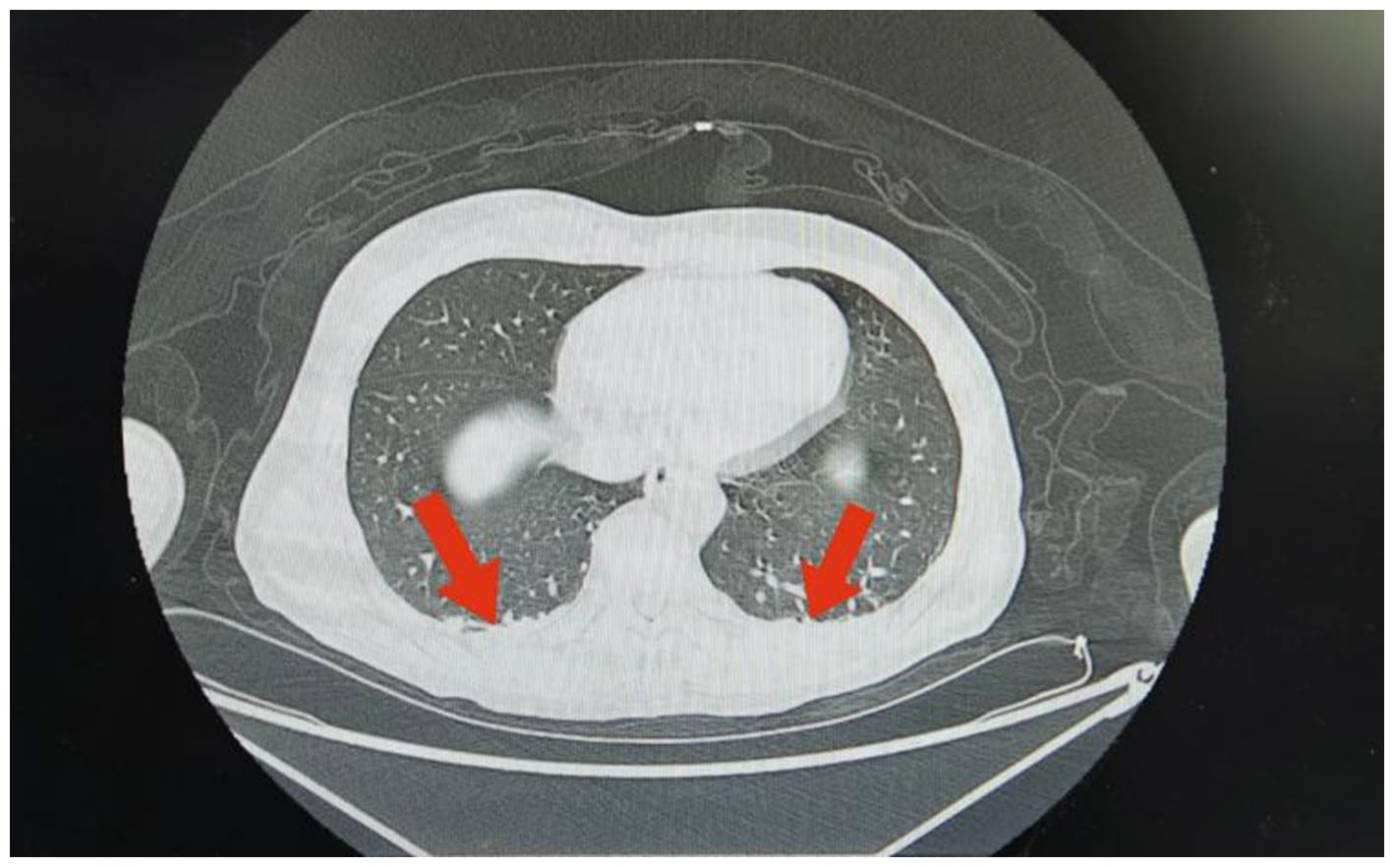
Imaging findings in Case 1: Chest CT shows a small area of inflammation or exudation in the left lung.
